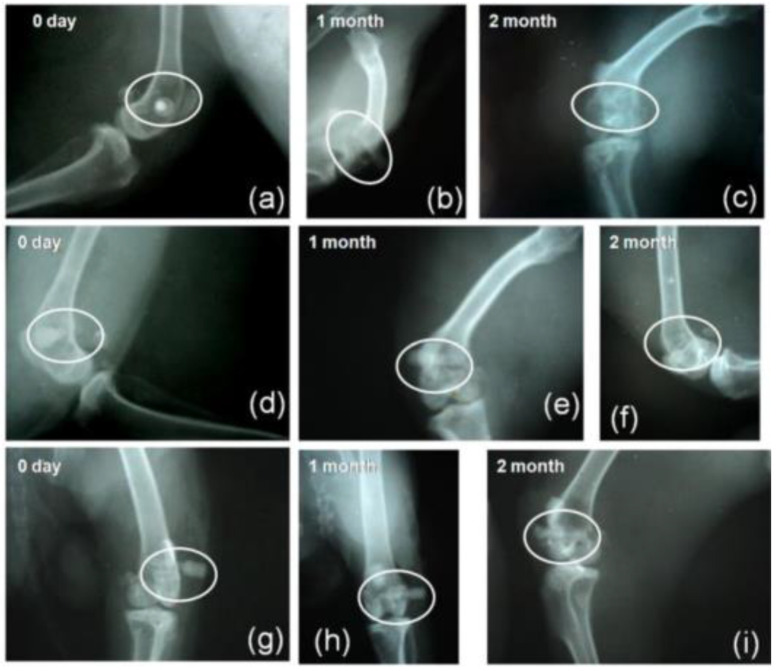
Radiographs of BM (a–c), BMH (d–f) and BMG (g–i) placed in bone immediately after implantation (day 0) and 1 month and 2 months after surgery [156].
